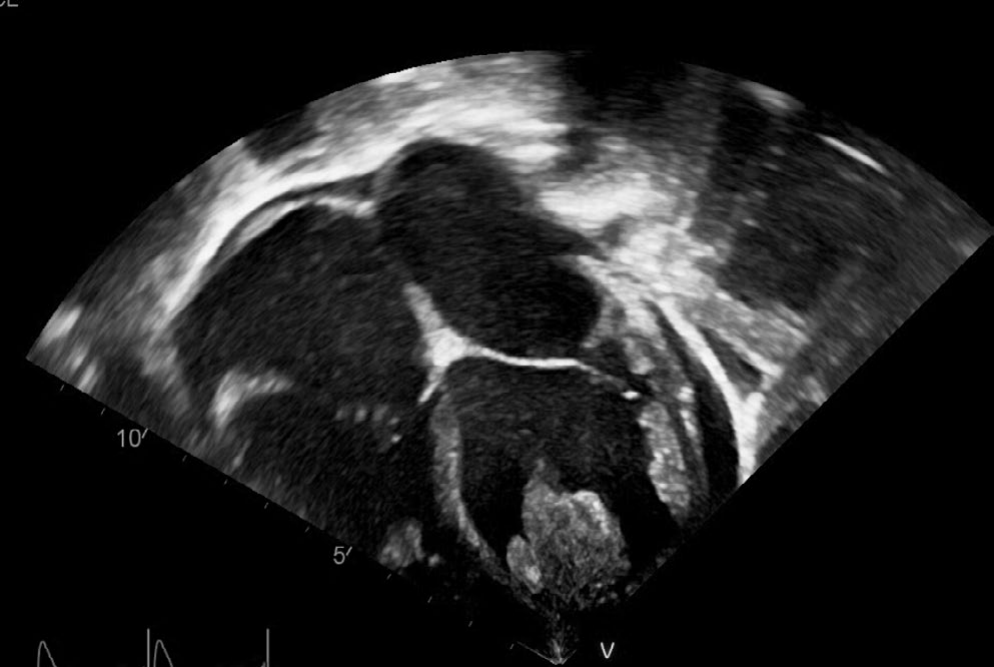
Echocardiography of Case 4 showing Large thrombus seen in the left ventricle, attached to the anterior septum and anterior wall (wide base) with multiple lobes and some mobile extensions
